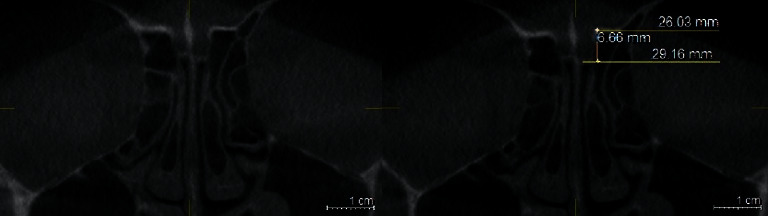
Coronal plan of CBCT demonstrating type 2 Keros classification on the left side.
